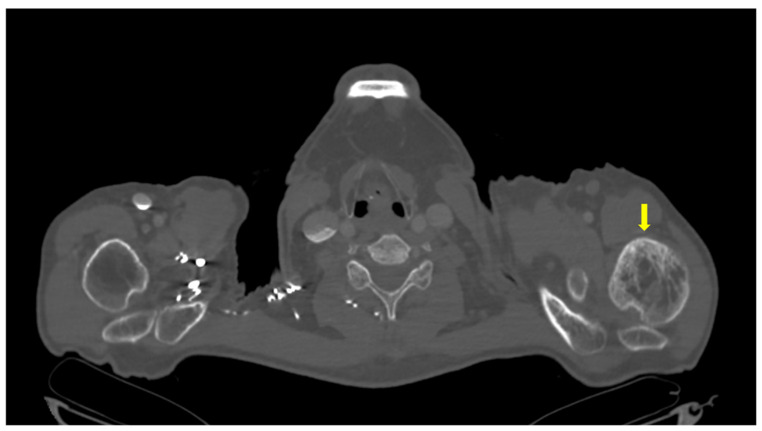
Contrast CT showing Paget’s disease of bone lesion at the left humerus (yellow arrow).
